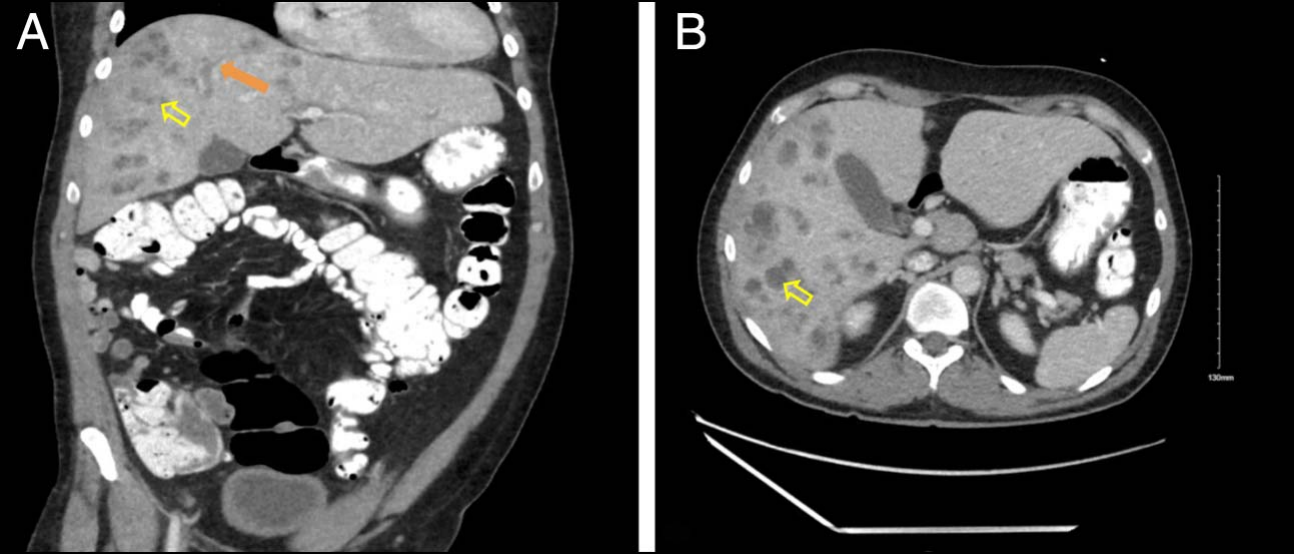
Computed tomography of the abdomen with contrast coronal section (A) and axial section (B) shows multiple ill-defined right hepatic hypodensities (yellow open arrows) and intrahepatic duct dilation (orange arrow).
